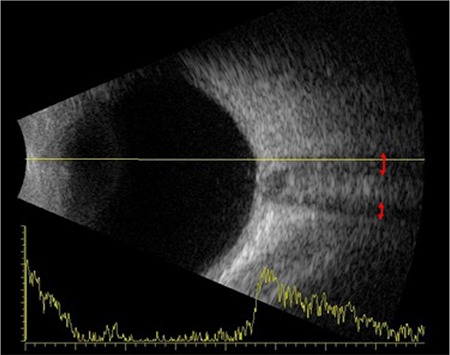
B-scan ultrasonography of the right eye shows abnormally increased optic nerve sheath diameter (red double-headed arrows)
